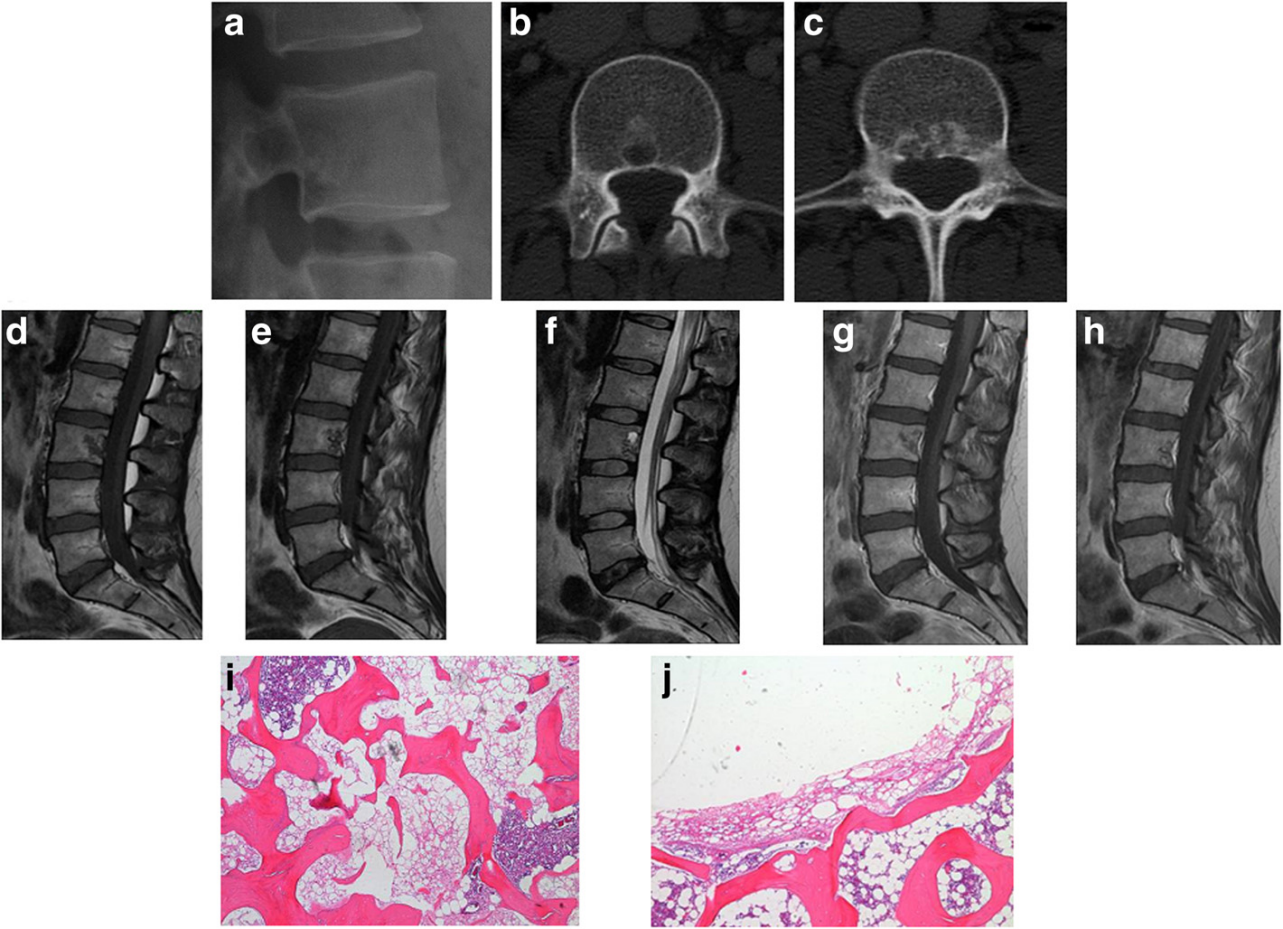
Patient 1, a 33 year-old woman. Images demonstrate radiodensity (sclerosis) (radiograph, a) as well as lytic areas (CT, b and c). The lesion is heterogeneous on both T1- (d and e) and T2-weighted (f) sequences and takes up contrast moderately (g and h). The sclerotic aspect on imaging corresponds to classic areas of BNCT: permeative sheets of adipocyte-like tumor cells associated with bone sclerosis and mixed with normal bone marrow islands (i). The round lytic area on imaging (c) corresponds to a centrally emptied notochordal cell lesion without evidence of chordoma at the periphery of the lesion (j). The cystic nature of this lesion is best seen on T2-weighted imaging (f).
